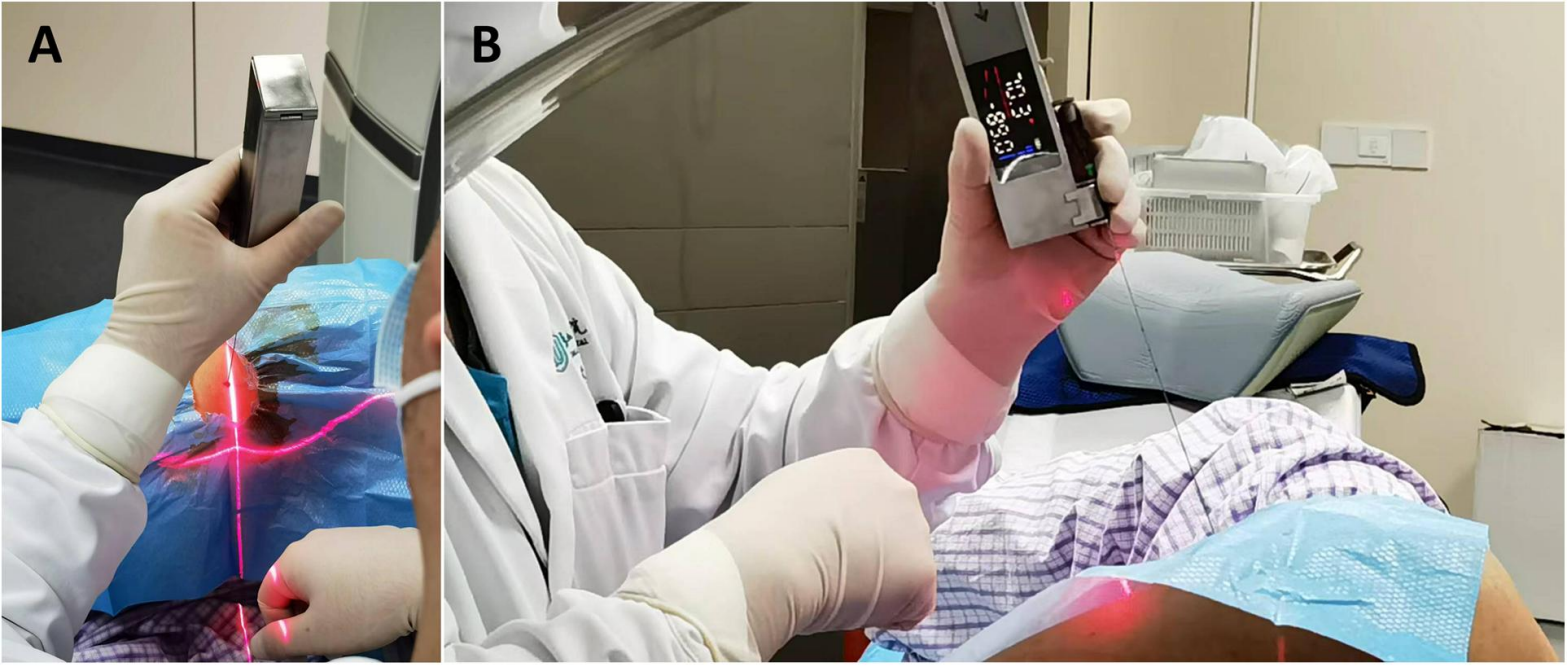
Perform the puncture under the guidance of the laser angle guider. The angle of the guider is adjusted to make the horizontal line of the laser coincide with the horizontal line corresponding to the puncture point and to make the cross light of the laser parallel to the longitudinal and transverse axes of the CT bed respectively.The angle of the guider is adjusted to make the X*Y* axis (parallel to the patient's horizontal plane) displayed on the laser angle meter be the intended needle insertion angle, and the Z axis (parallel to the patient's sagittal plane) angle be 0 degrees.
